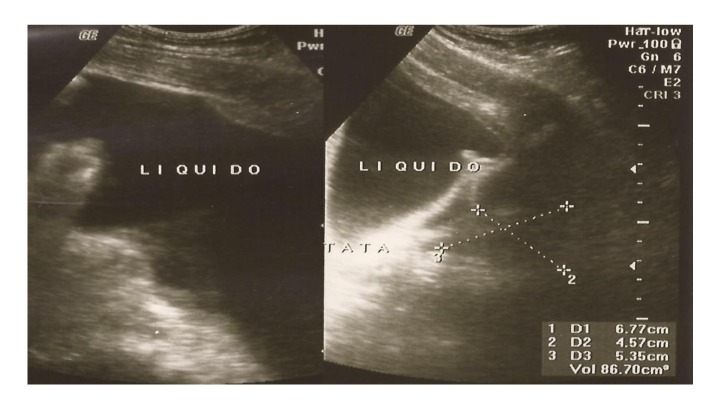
Abdominal ultrasound with a large volume of ascitic fluid in the peritoneal cavity.
